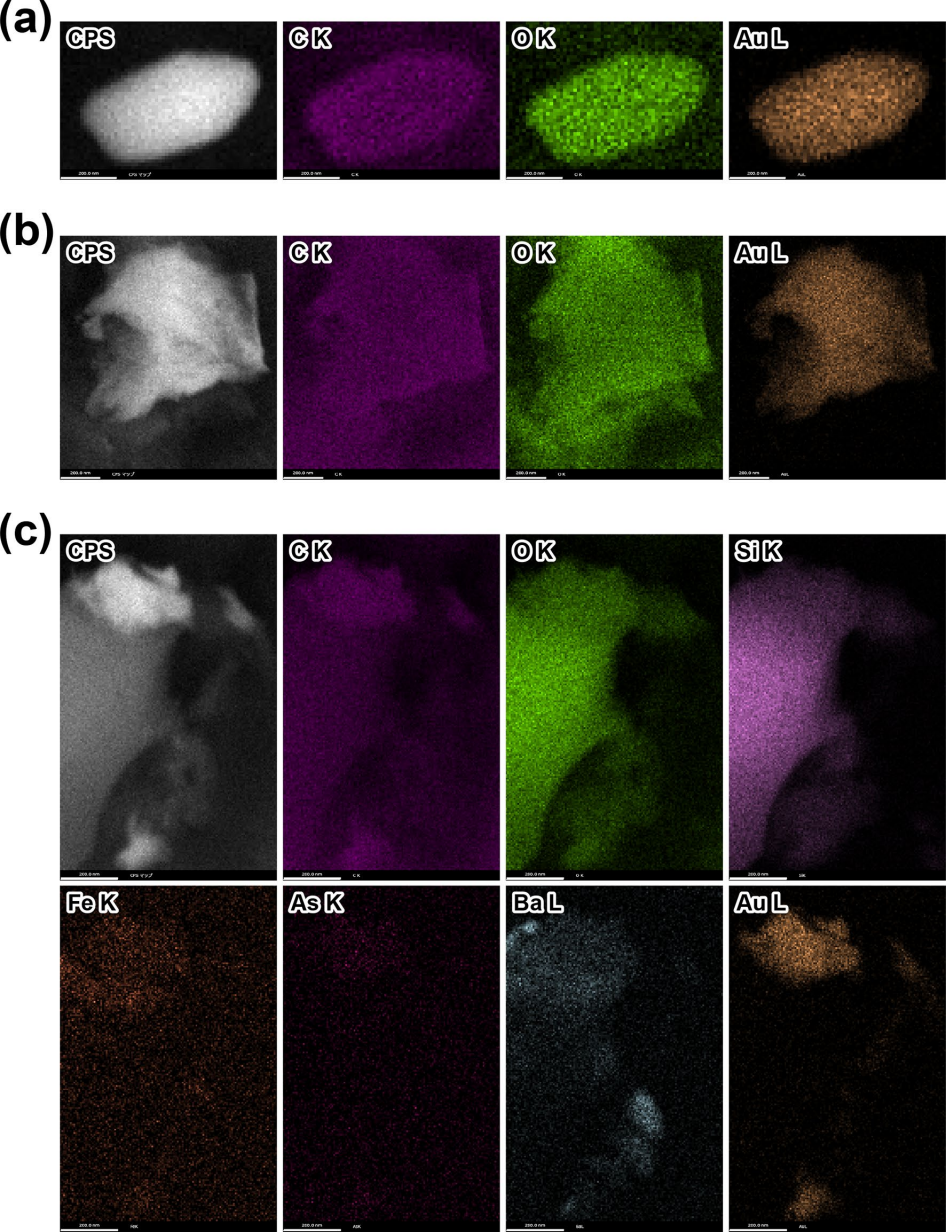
SEM–EDS elemental mappings. (a–c) Mapping results for the Au-rich grains shown in Fig. 3c–e. The EDS elemental maps were obtained from the L lines for Ba and Au and from the K lines for other elements. X-ray counts per second (CPS) maps were also obtained. White scale bars indicate 200 nm.
